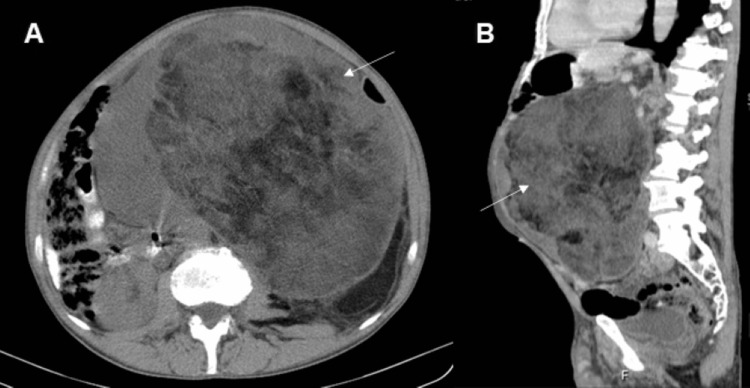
Computed tomography scan of the abdomen and pelvis showing an 18 × 25 cm left retroperitoneal mass: axial (A) and sagittal (B) view (white arrow).
